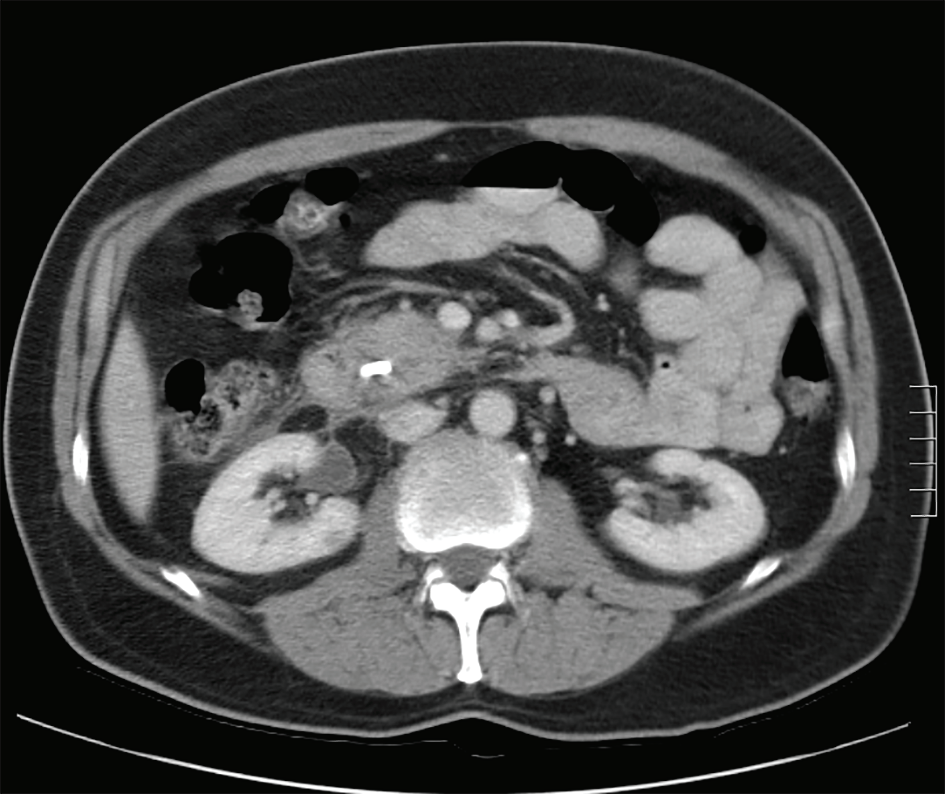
Initial CT scan demonstrating pancreatic head mass.
